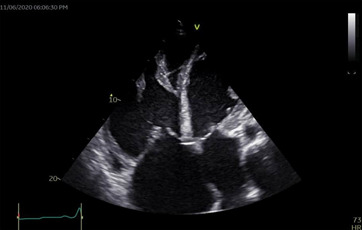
two-dimensional echocardiography (apical four-chamber view) showing large secundum ASD of 27mm, mild dilation of the left atrium, severe right ventricular and right atrial dilation
